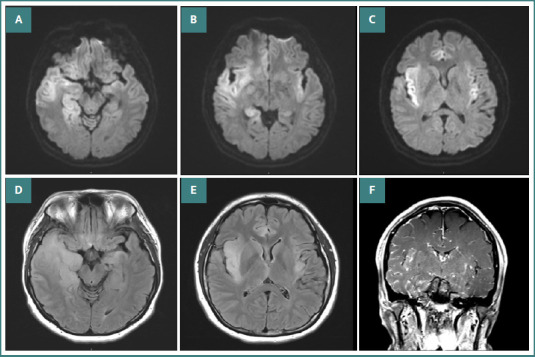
Brain MRI diffusion-weighted images. A–C indicated a diffuse cortical diffusion restriction lesion centered on the insular region. Brain MRI FLAIR images; D–E also demonstrated this finding. Additionally, brain MRI with gadolinium enhancement (F) suggested focal cerebral edema and a diffuse inflammatory lesion in the right temporal and left insular regions.
